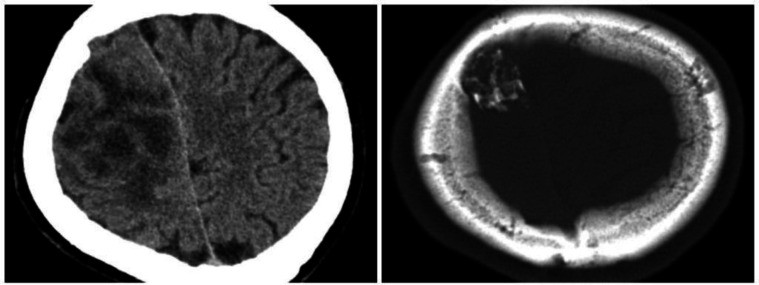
Preoperative non-contrast computed tomography revealed a heterodense mass with a calcified component in the right frontoparietal region
